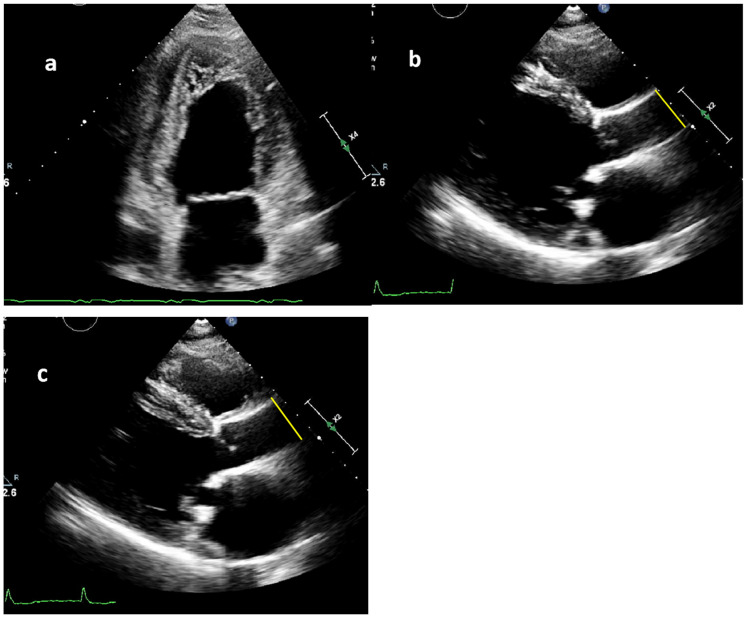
(a–c): Measuring technique of the systolic and diastolic ascending aortic diameters. (a) The two-chamber view of the left ventricle and left atrium in a noncompaction cardiomyopathy patient. (b,c)The transthoracic echocardiographic images of a 51-year-old male with dilated cardiomyopathy (DCM). He could only walk 200 m at presentation. (b) The yellow line presents the measurement of the diastolic aortic diameter (26 mm). (c) The yellow line presents the measurement of the systolic aortic diameter (29 mm).
